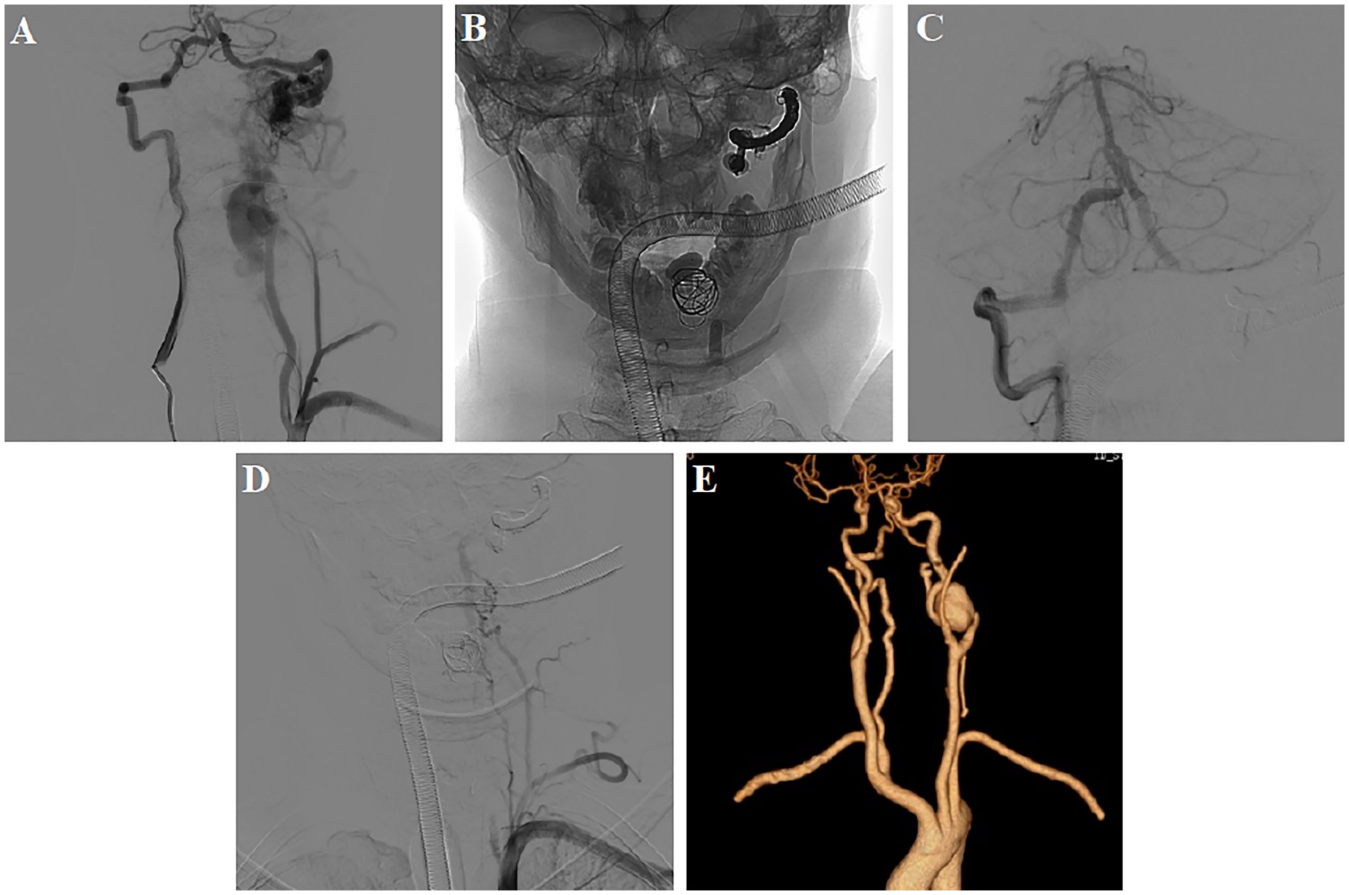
First postoperative DSA and CTA examination. (A) DSA demonstrated multiple high-flow arteriovenous fistulae at the left CVJ (fistula A) and the C3–C4 level (fistula B); (B–D) Balloon combined with a coil and Onyx embolization, leaving an anastomotic muscular branch backflow vertebral artery supplying blood to fistula B; (E) CTA showed the disappearance of abnormal blood vessels in the left neck.
